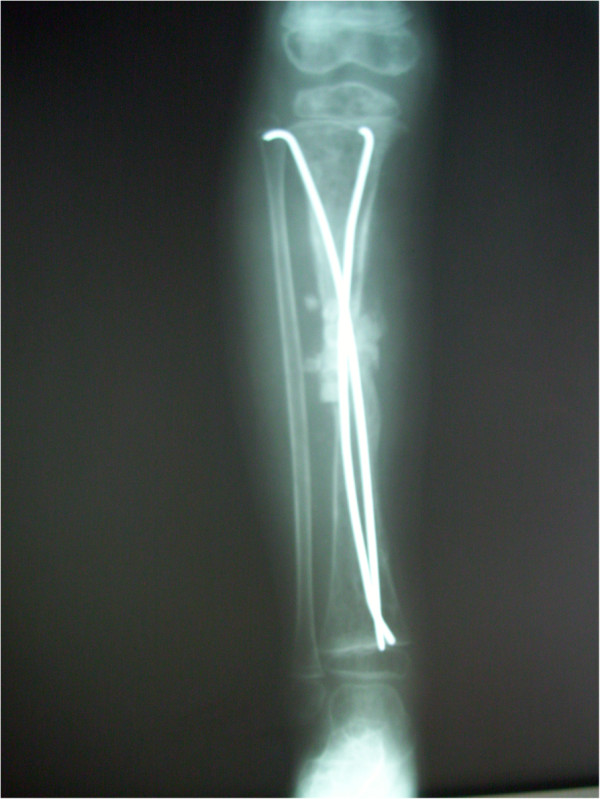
Anteroposterior and lateral radiographs of the patient’s left tibia after intramedullary nailing done for pathologic fracture.
